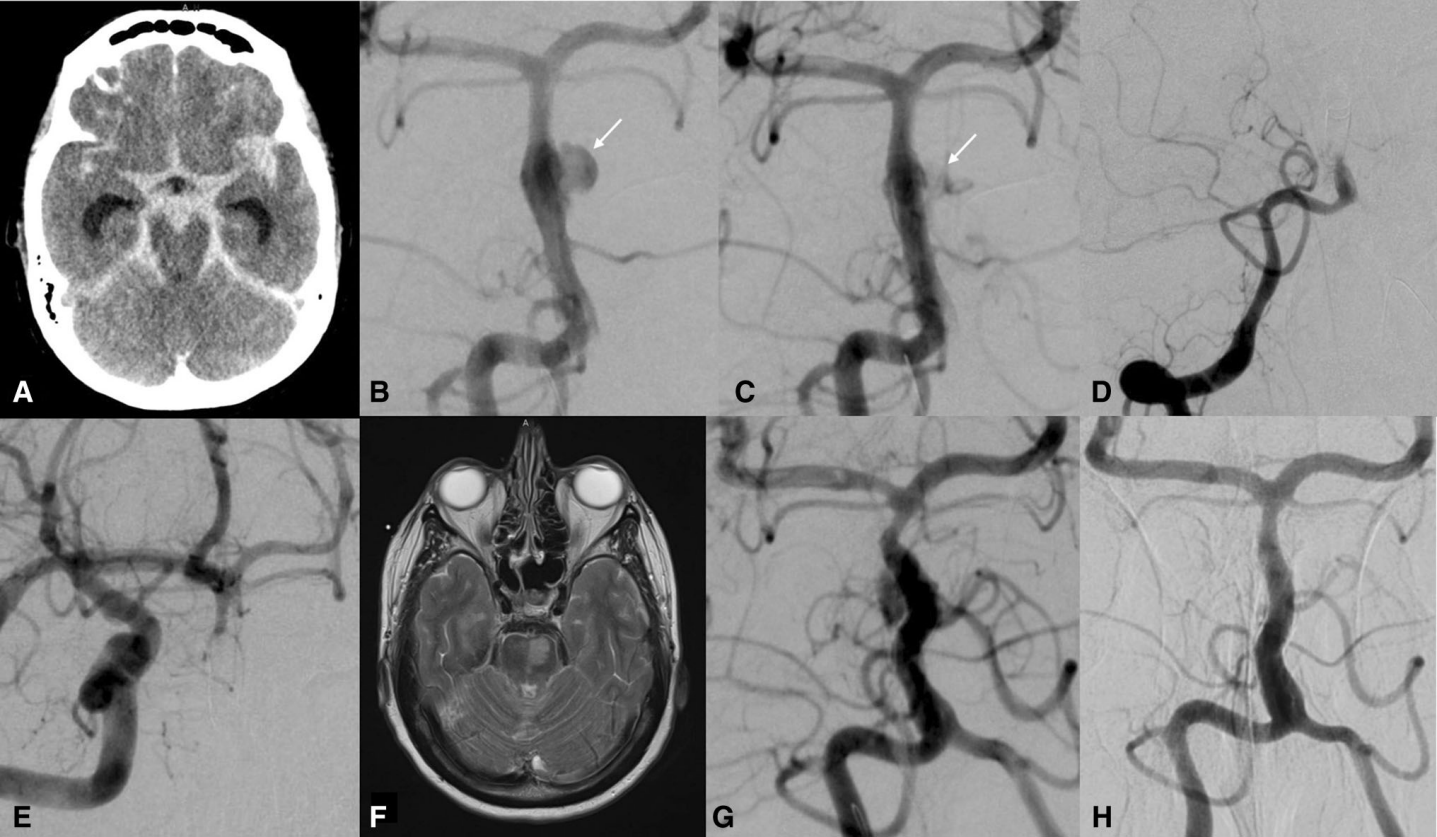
A 58-year-old female presented with a sudden headache after impact with the trunk lid, followed by deterioration with nausea, vomiting, and unconsciousness (Patient #7). A cranial CT scan obtained on the day of admission revealed diffuse subarachnoid hemorrhage (Fisher grade 4) and hydrocephalus (A). Posterior–anterior arteriogram obtained with a right vertebral artery injection showing a fusiform dissecting aneurysm of the BA with the formation of a pseudoaneurysm (arrow) (B). Endovascular treatment was performed on day 1 after bleeding. Multiplate and VerifyNow tests confirmed sufficient platelet inhibition after premedication with 1000 mg ASA IV. Posterior–anterior projection obtained immediately after telescopic deployment of 5 p48MW HPC FDS (C). Insufficient platelet inhibition by ASA after treatment resulted in in-stent thrombosis on day 3 (D). The distal part of the BA was retrogradely perfused via the posterior communicating artery (E). T2WI MRI is showing a left pontine infarction due to the thrombosis of a pontine branch together with the dissecting aneurysm (F). Recanalization of the BA, including the aneurysm, was observed after the switch to DAPT plus eptifibatide IV (G). The patient was retreated with two p48MW HPC implanted distally before discharge. The most recent follow-up angiography performed 9 months after treatment shows complete exclusion of the pseudoaneurysm with total reconstruction of the previously dissected BA and patency of the FDS (H)
